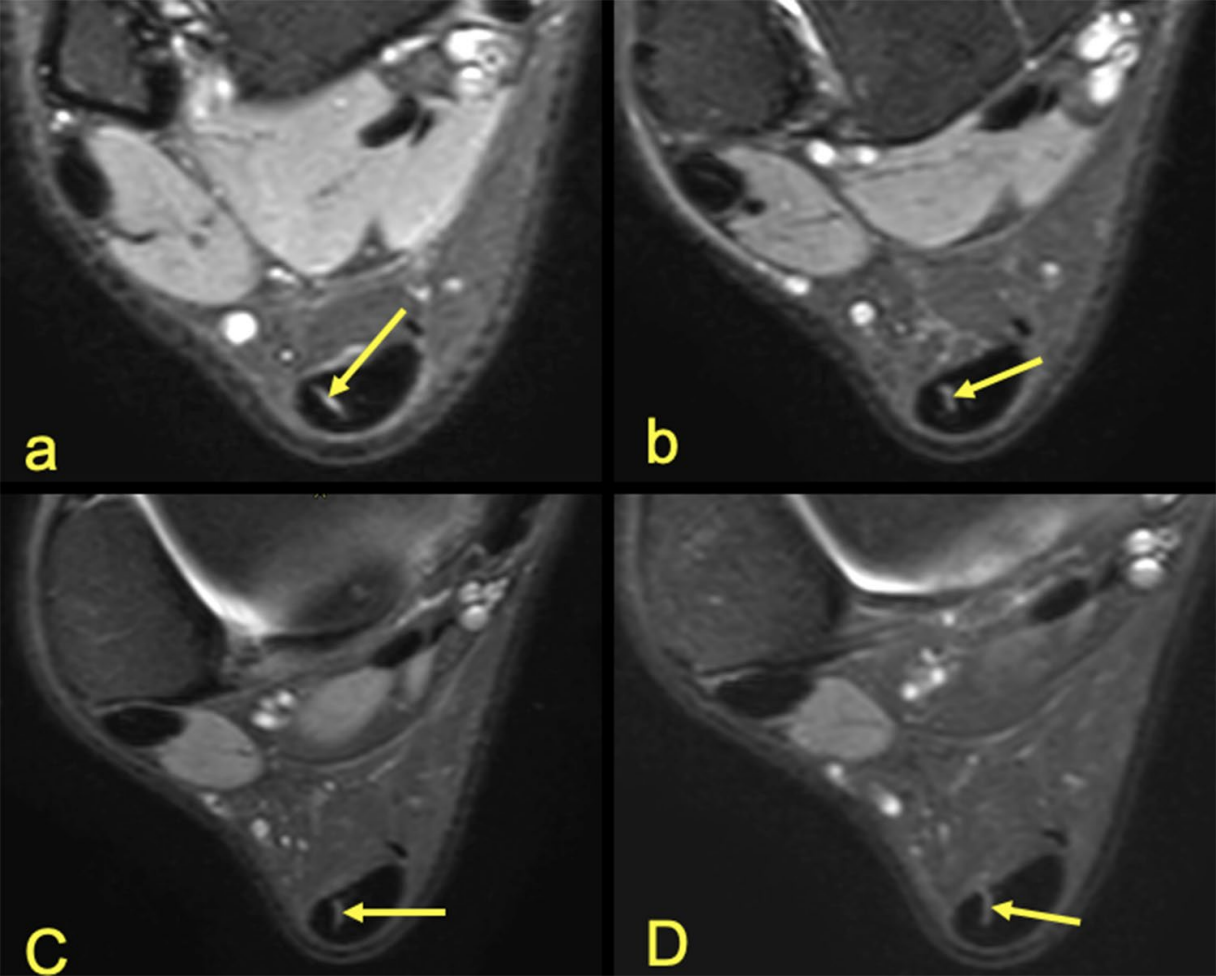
MRI right ankle, PD FS at the level of the talus. (a)—The highest section, (d)—the lowest section. A 42-year-old male with ankle sprain without trauma. The arrow marks a narrow septation between the subtendon from the lateral head of the gastrocnemius muscle and the other subtendons of the Achilles tendon.
